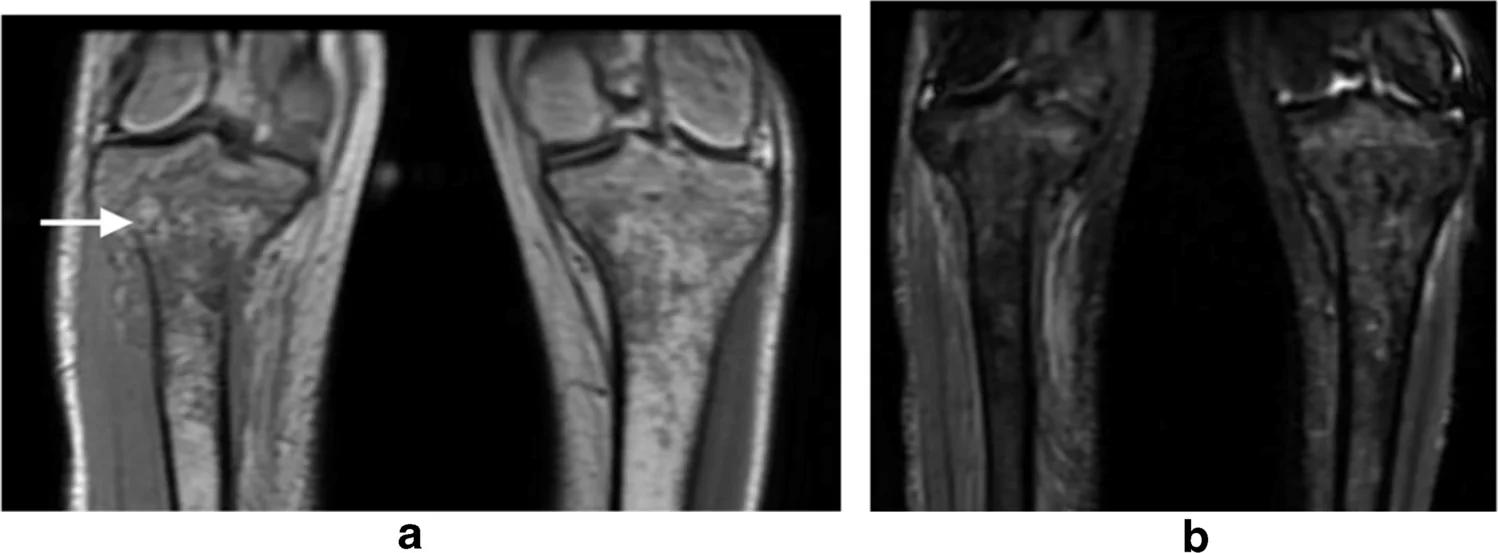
Image obtained in 20-year-old man with acute lymphoblastic leukemia involving both tibias. a Coronal T1 MR imaging of the knees shows diffuse heterogeneity of the bone marrow within the bilateral proximal tibias, as well as within the tibias bilaterally, manifested as areas of low T1 signal (solid arrow). b Coronal T2 MR imaging of the knees shows patchy areas of high T2 signal. These findings likely represent marrow infiltrative changes associated with leukemia and possible superimposed bone infarcts
